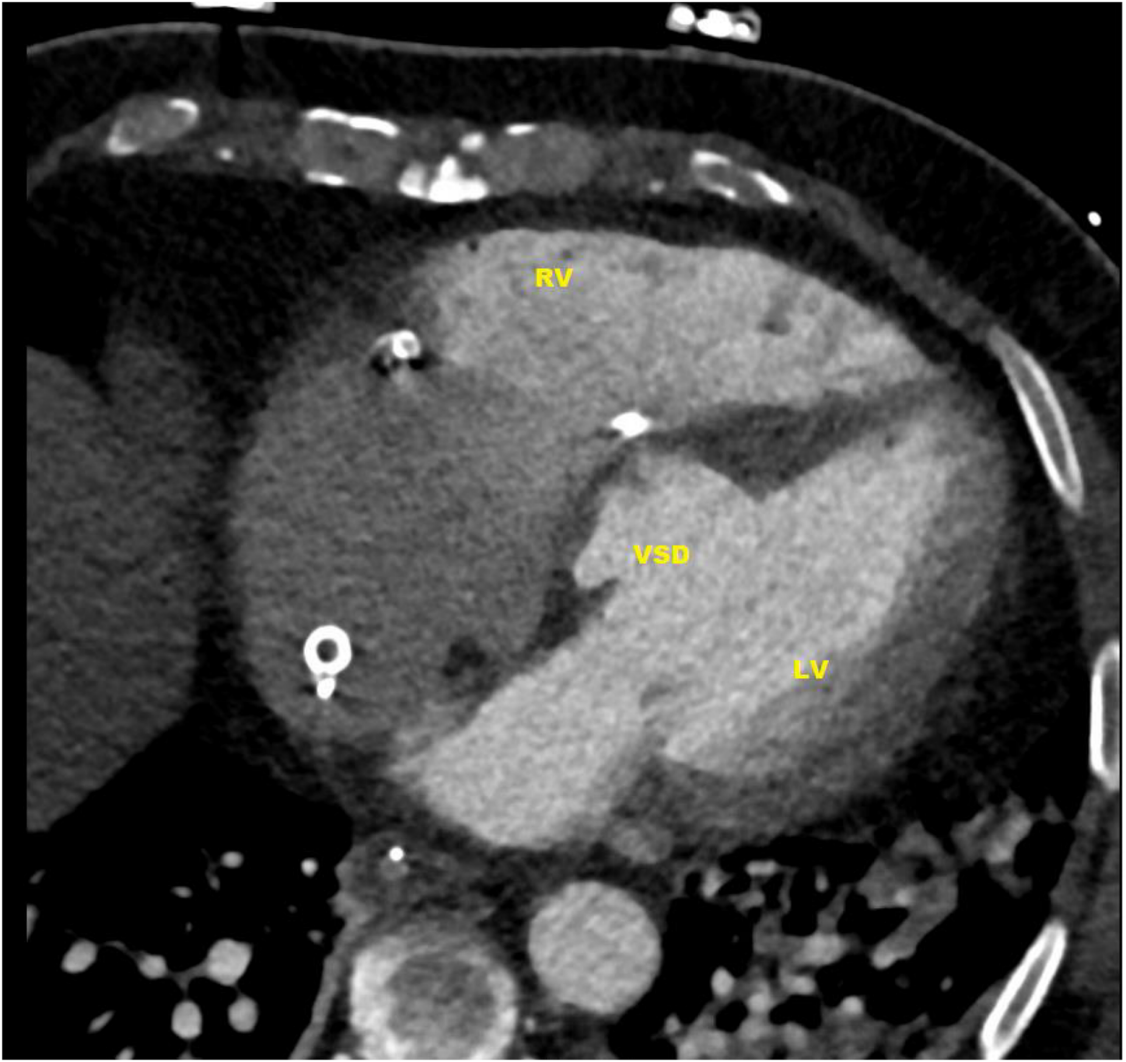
CT-scan: ventricular septal defect (VSD) directly under the valvular plane, volume-loaded left and right ventricle (LV, RV).
